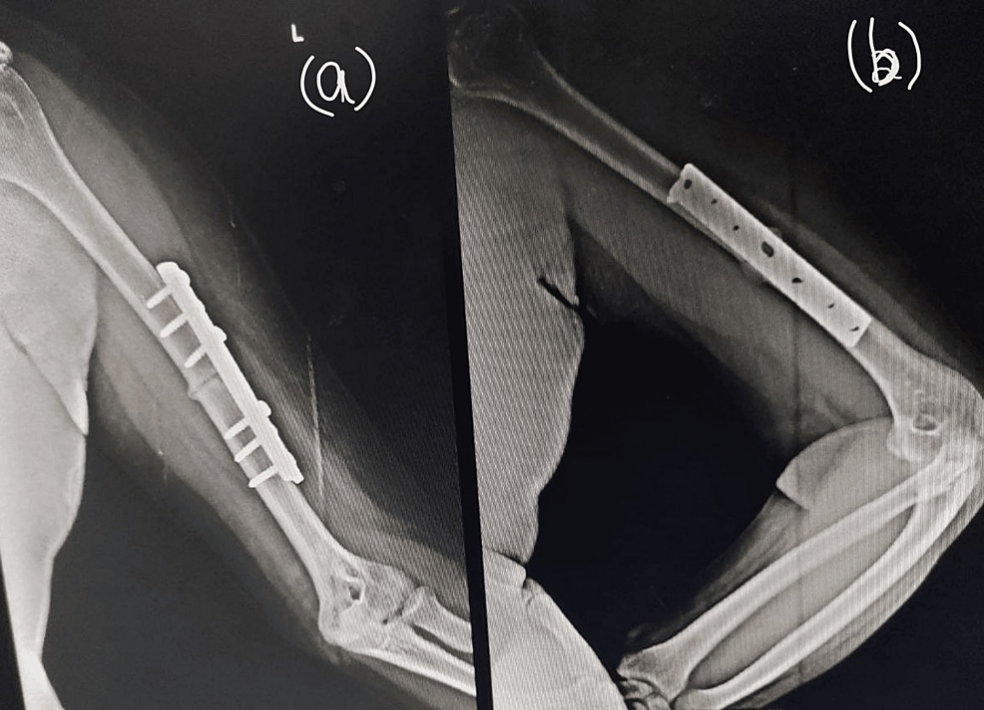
Callus formation seven weeks after initiation of teriparatide (19 weeks from the time of internal fixation) Radiograph showing left humerus (a) anteroposterior view, (b) lateral view
